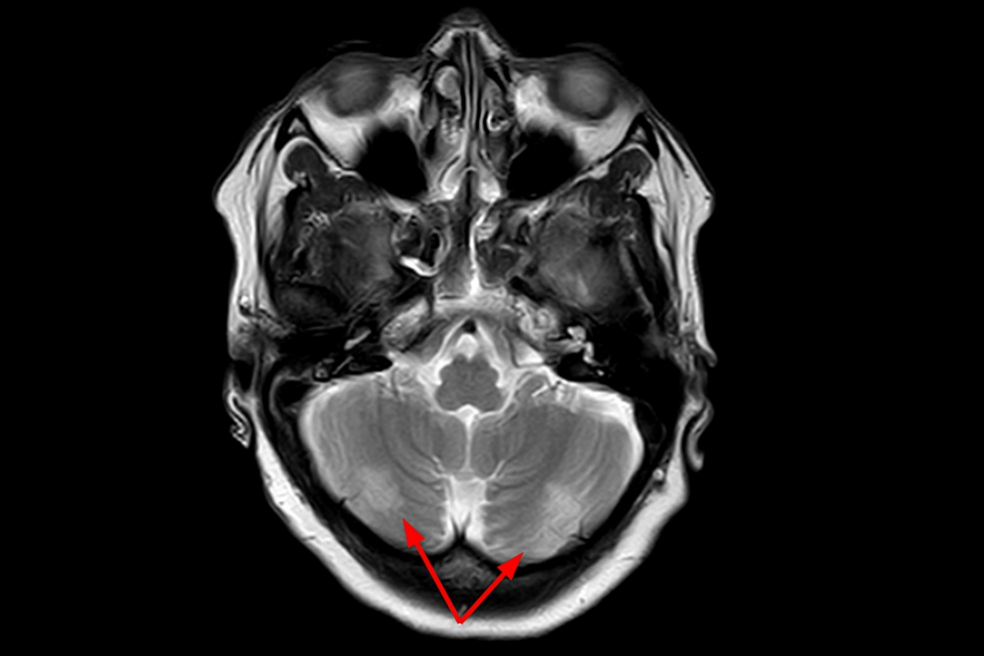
T2-weighted MRI of the brain-axial section showing subtle focal patchy hyperintensities in bilateral cerebellar hemispheres (arrows)
